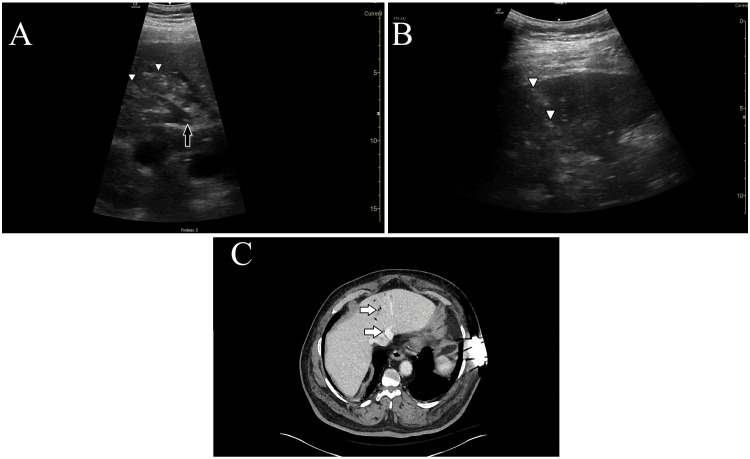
Case 3 - POCUS images and CT A: Narrow-sector B-mode image shows hyperechoic foci of gas (white arrowheads) within the liver, with flowing bubbles (black arrow) visible in the portal vein. B: Full-sector B-mode image shows hyperechoic foci of gas (white arrowheads) within the liver parenchyma. C: CT image demonstrates gas (white arrows) within the portal veins. B-mode: brightness mode; POCUS: point-of-care ultrasound; CT: computed tomography
